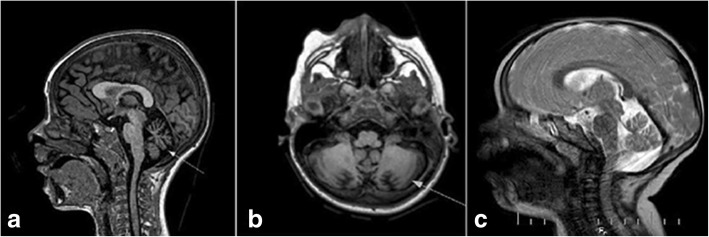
Patient A. Follow-up MRI at 47 months: a) Sagittal T1-weighted image showing cerebellar atrophy (arrow); b) Axial FLAIR image showing hyperintensity of cerebellar white matter and dentate nuclei (arrow). Patient B. MRI at 24 months: c) Sagittal T2-weighted image showing megacisterna magna and atrophy of vermis
